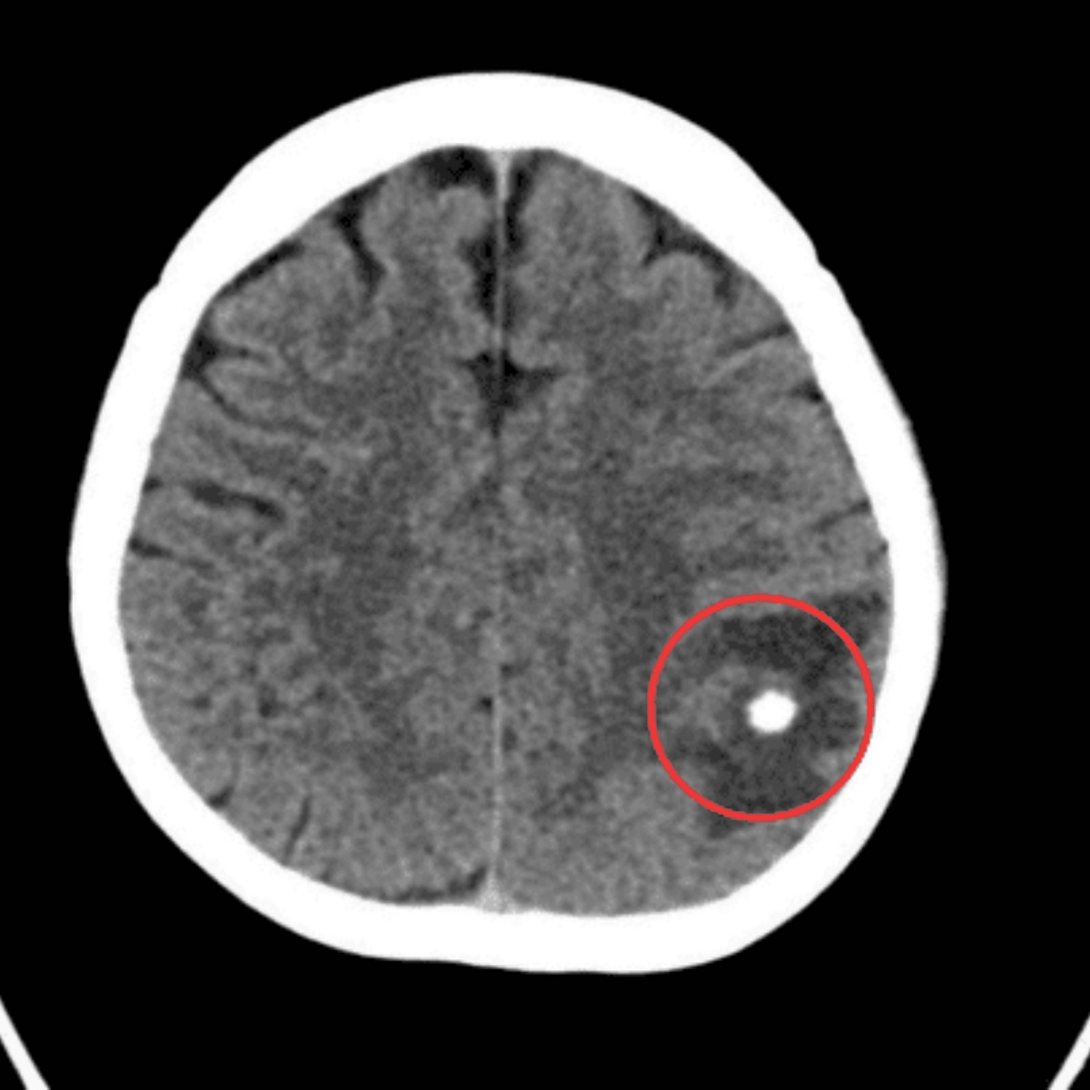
Non-contrast CT brain showing a hyperdense lesion in the left parietal lobe (red circle) with minimal surrounding edema, likely representing a calcified granuloma.
